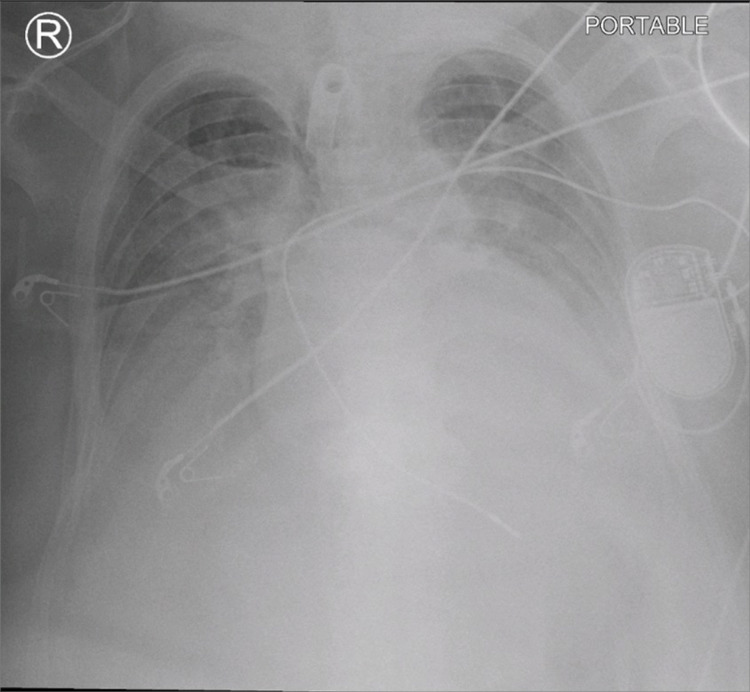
Chest X-ray revealing severe acute respiratory distress syndrome A pacemaker was inserted for arrhythmia.
